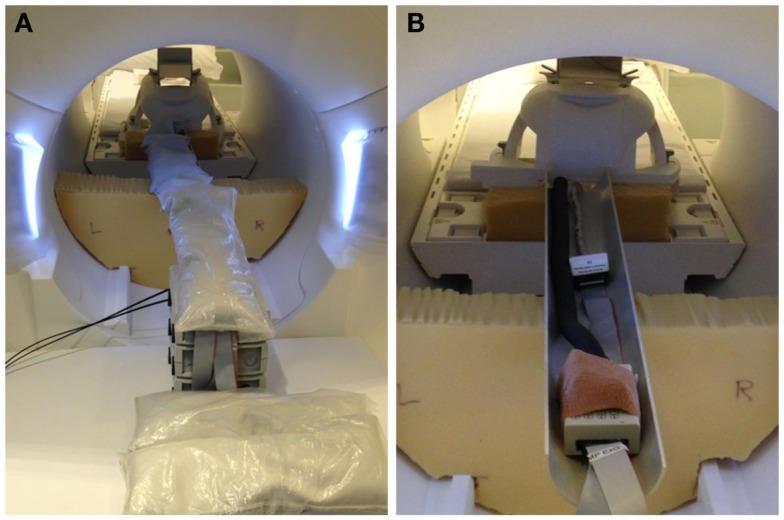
Photographs of inside-MRI-scanner EEG equipment layout similar to that utilized in the present experiment. To obtain a clear view, these photographs were taken in our mock-scanner; in our real scanner, we use a head coil that permits entry of the cables directly from the rear. (A) The EEG amplifier and peripheral signal amplifier are both placed outside the scanner bore and the cable connecting the EEG cap to the amplifiers fixed in place in the center of the bore using plastic piping covered with sandbags, suspended on pieces of thick foam padding. (B) View of the cables with the sandbags removed.
